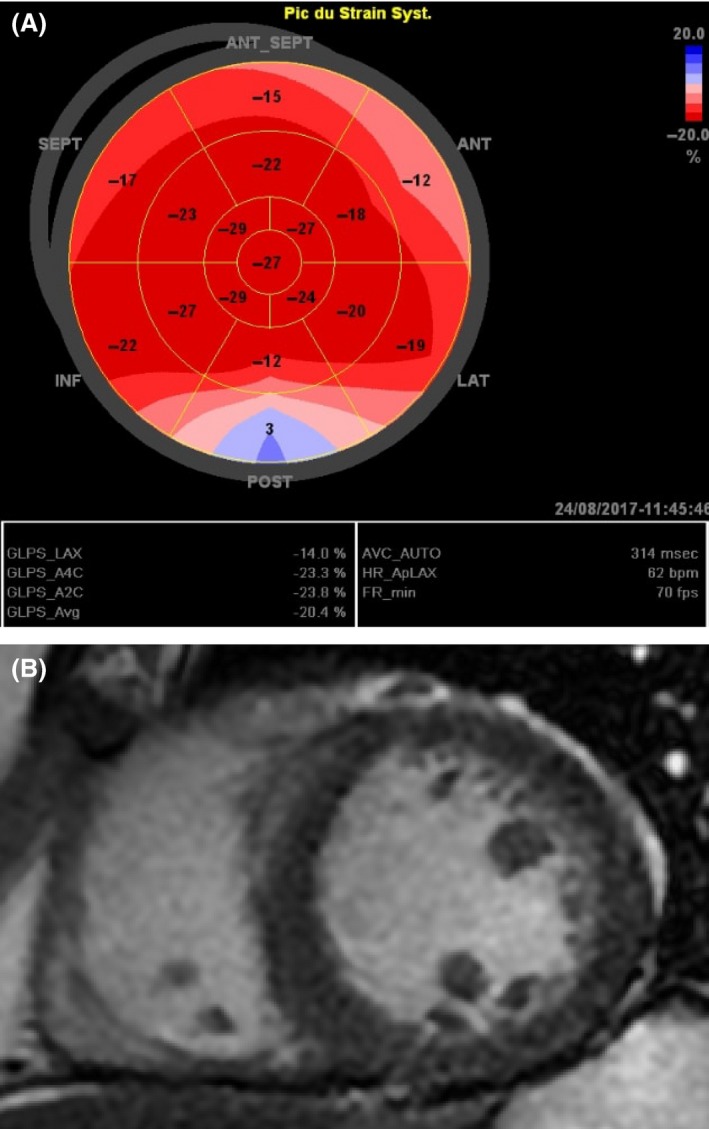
Panel A: Bull's eye strain showing a normal GLS at −20.4%, with a little bit altered in the posterior and postero‐lateral segments of the myocardium. Panel B: Cardiac magnetic resonance imaging (CMR) revealing a LGE of the subepicardial layers of the lateral wall of the left ventricle in patient *n*°1.
